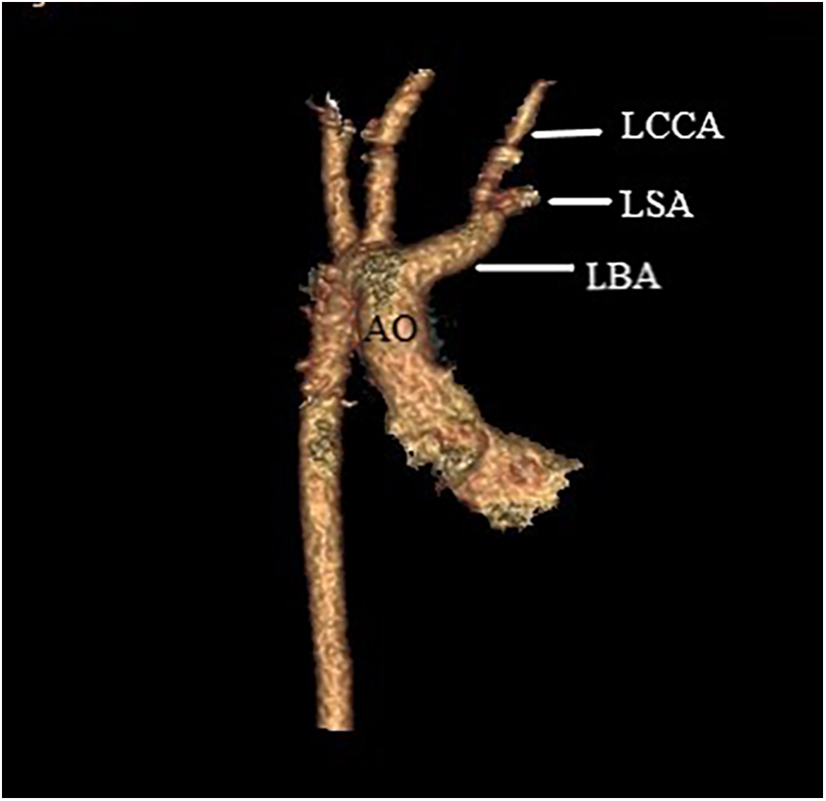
Postoperative computed tomographic angiography showed that the reconstructed LBA was patent. AO, aorta; LCCA, left common carotid artery; LSA, left subclavian artery; LBA, left brachiocephalic artery.
